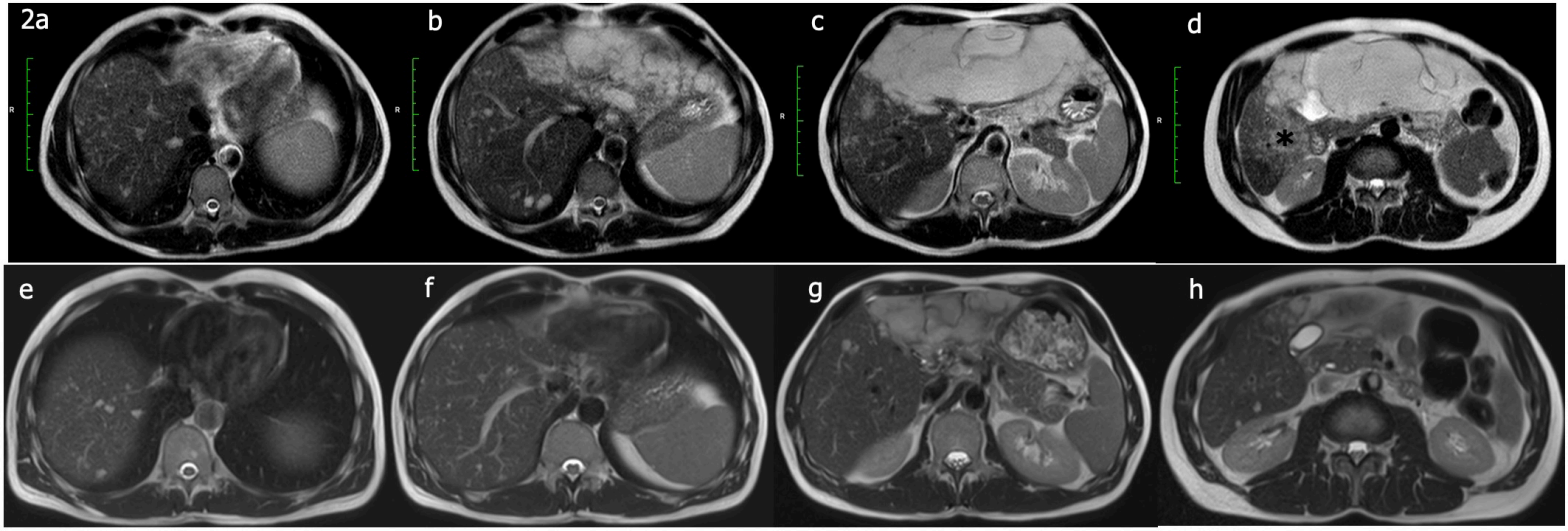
(a–d) Giant hemangioma extending from the left lobe to segment 6; hemangiomatous changes were evident in the adjacent areas (*). (e–h) MRI taken 4 years after a single TACE session. Marked regression is evident.
